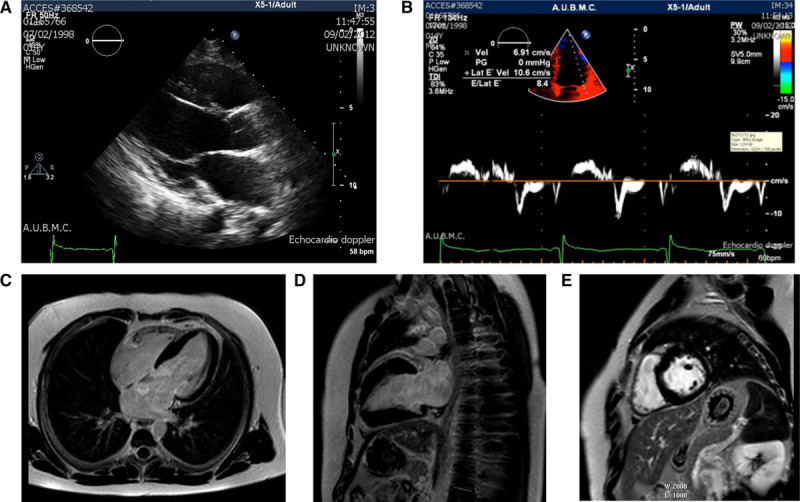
Advanced cardiac imaging of genotype-positive but phenotype-negative patient. The 13-y old man (patient III-18 from DH232-A) is asymptomatic and carries the p.Arg21Cys mutation. Cardiac imaging reveals the absence of hypertrophy on echocardiography (A); Doppler tissue myocardial velocities at the lateral aspect of the mitral annulus (B) show reduced Ea and S velocities, suggesting early systolic and diastolic myocardial dysfunction. Cardiac magnetic resonance images in end diastole (C–E) show a relatively asymmetrical wall thickening of the basal and mid-anteroseptal and inferoseptal walls as compared with the lateral wall (10–11 vs 6–7 mm). The wall thickness per se is within normal, and the left ventricular mass total is also normal. There is uniform nulling of the myocardium on delayed imaging post-gadolinium, including the area of maximal thickening. No evidence of scar or fibrosis or infiltrative disease was seen.
